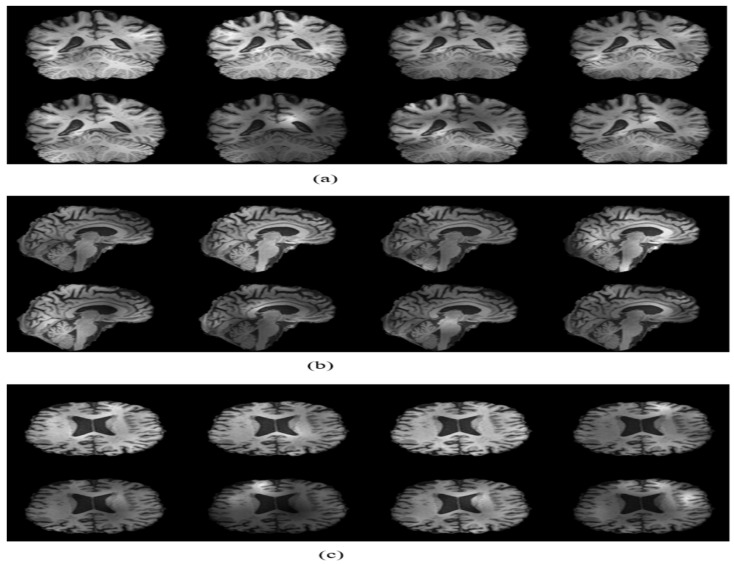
Grad-CAM visualizations overlaid on multi-view MRI slices. (a) Coronal, (b) sagittal, and (c) axial Grad-CAM maps generated by the proposed model.
